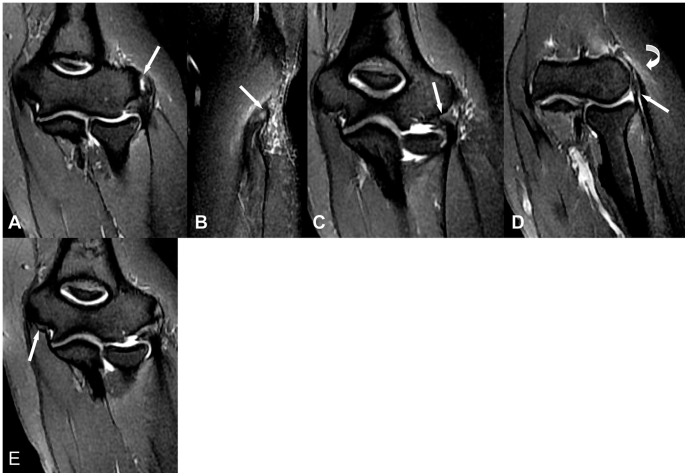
A 36-year-old female with left elbow pain about 1 month. (A,B) Coronal fat-suppressed FSE T2-weighted image shows abnormal fluid signal intensity affecting about 50% of the CET thickness (white arrow), demonstrating moderate injury. (C) Coronal fat-suppressed FSE T2-weighted image shows thickening and abnormal mildly increased signal intensity within the LUCL origin (white arrow), which suggests mild injury. (D) Coronal fat-suppressed FSE T2WI shows thickening and mildly increase signal intensity within the RCL (white arrow), demonstrating mild injury. Intramuscular edema shows as high-signal-intensity focus is seen within the extensor carpi muscle (white curve arrow ). (E) Coronal fat-suppressed FSE T2-weithted image shows mildly increased signal intensity within the proximal MCL (white arrow), a finding suggestive of mild injury.
